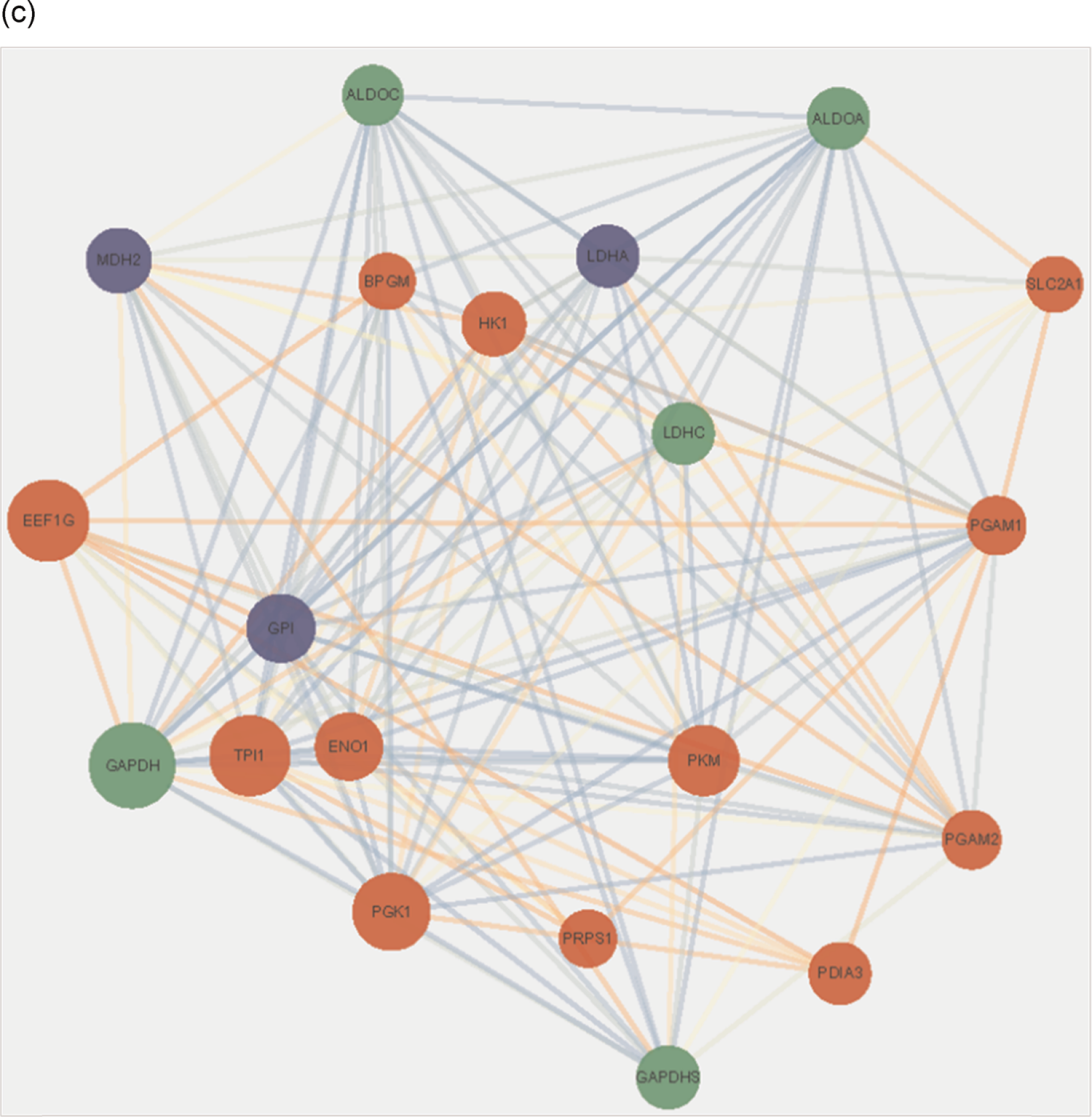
To reveal the crosstalk between phosphoproteome and ubiquitylome in more detail, the protein–protein interaction network based on phosphorylation and ubiquitination proteins was established. The global overview of protein–protein interaction network among phosphorylation and ubiquitination proteins were obtained using the STRING database and Cytoscape software (Figure S1), and two highly interconnected clusters were retrieved (Figure 5b,c). We observed that both phosphorylation and ubiquitination proteins participated in the proteasome and glycolysis pathways. Eight proteins (proteasome 26S subunit ATPase 1 [PSMC1], proteasome subunit alpha type‐4 [PSMA4], 26S proteasome non‐ATPase regulatory subunit 4 [PSMD4], proteasome activator complex subunit 4 [PSME4], T‐complex protein 1 subunit gamma [CCT3], T‐complex protein 1 subunit theta [CCT8], valosin‐containing protein (VCP), and ornithine decarboxylase antizyme 3 [OAZ3]) and five proteins (glyceraldehyde‐3‐phosphate dehydrogenase [GAPDH], glyceraldehyde‐3‐phosphate dehydrogenase‐S [GAPDHS], fructose‐bisphosphate aldolase [ALDOC and ALDOA], and lactate dehydrogenase C [LDHC]) were both phosphorylated and ubiquitylated in proteasome and glycolysis clusters, respectively. These proteins may be of key importance in epididymal sperm and could be selected for further biological investigation.
